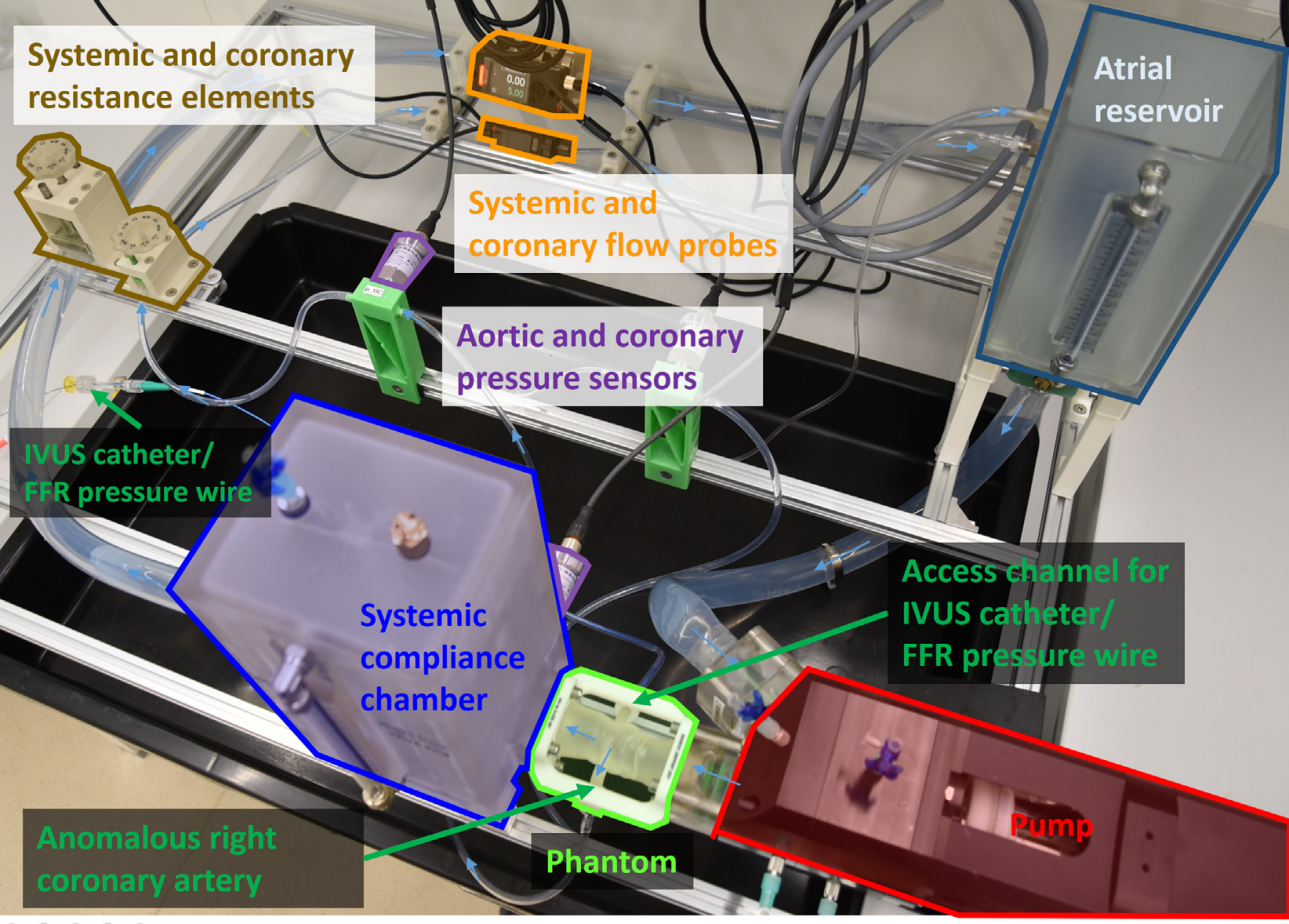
Flow Loop Setup Flow loop setup with the ability to assess FFR and IVUS anatomic deformation of the anomalous coronary artery in resting and stress conditions (mimicked physical exercise with increasing heart rate and blood pressure). FFR = fractional flow reserve; IVUS = intravascular ultrasound.
